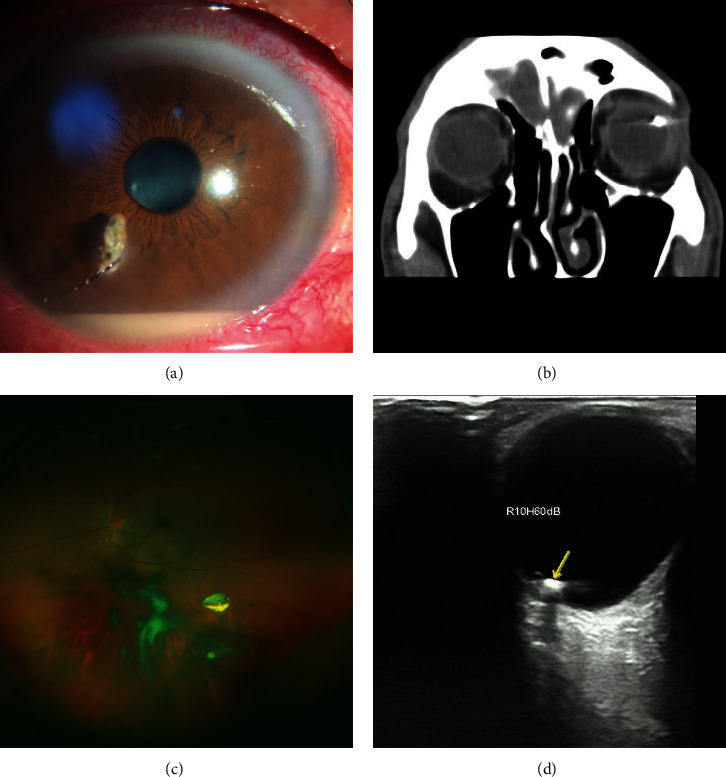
Clinical image of an intraocular foreign body. (a) An intraocular foreign body with an eyelash stuck at the cornea and penetrating the anterior chamber, and the presence of a hypopyon revealed that the patient has concomitant endophthalmitis. (b) Computerized tomography shows a metallic intraocular foreign body. (c) The fundus photo shows a metallic intraocular foreign body in the vitreous cavity with vitreous hemorrhage. (d) B-scan ultrasonography shows an intraocular foreign body with acoustic shadow.
